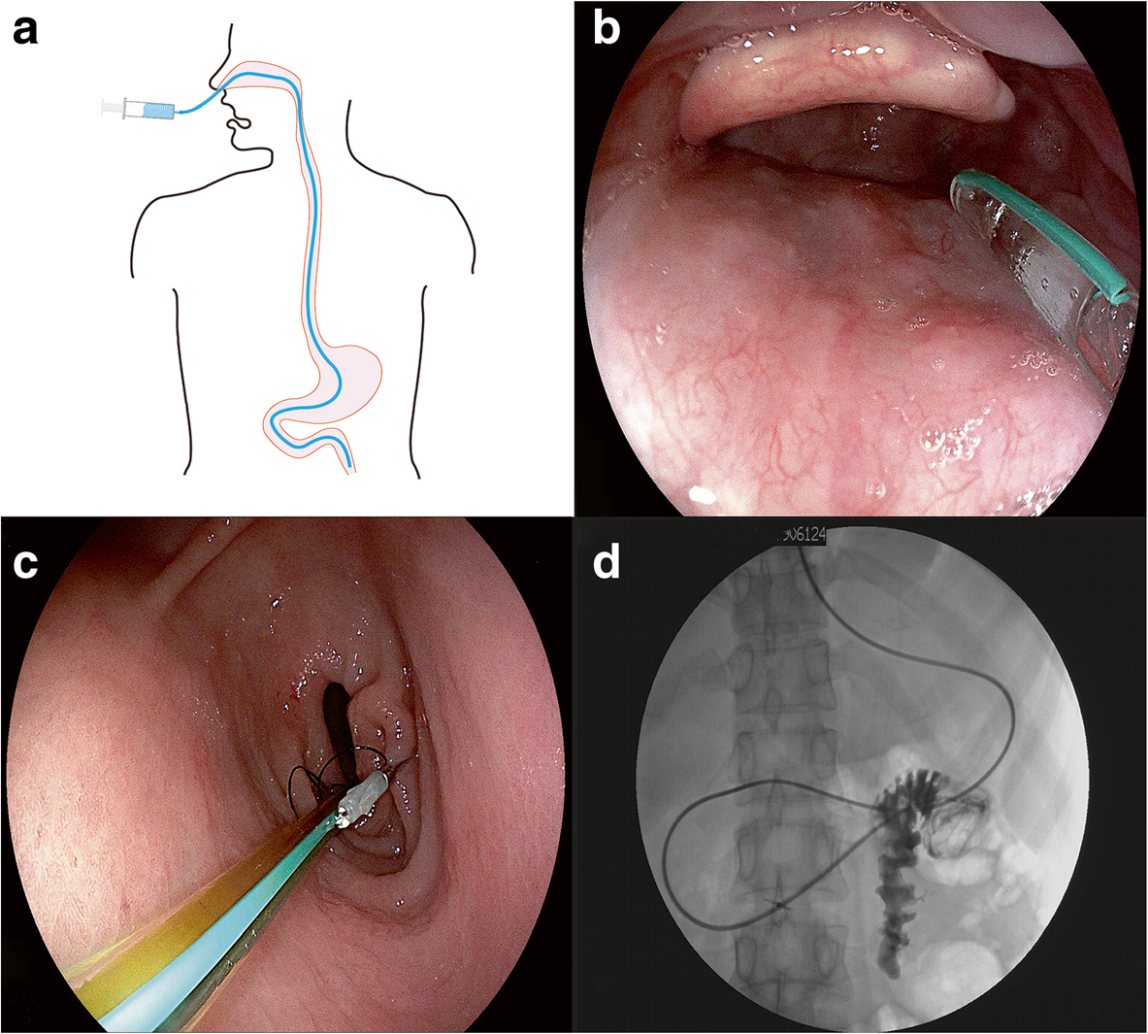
Concept and procedure of administration through mid-gut tubing. a The concept of administration through mid-gut tubing; b The endoscopic view at mouth cavity when the soft tube tip from nasal cavity was inserted into hypopharynx close to esophagus; c One tiny endoscopic clip was used for fixation of the tube at gastric antrum before the guide wire within the tube was removed out; d The location of mid-gut tube could be confirmed by X-Ray and contrast agent, but is not necessary using this TET technique and device
